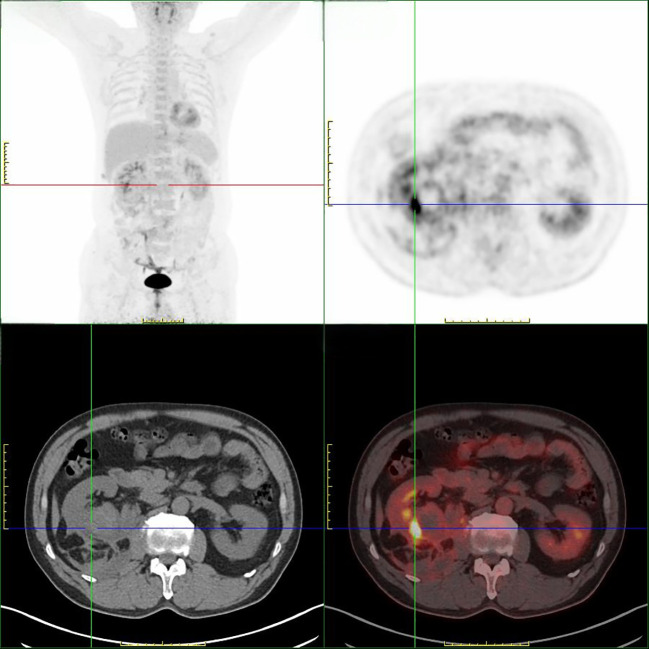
[18F]FDG PET/CT findings. Whole-body maximum-intensity-projection (MIP) image and regional axial CT, PET, and fused PET/CT images of the abdomen. The fused abdominal images show a focal area of increased [18F]FDG uptake posterior to the lower pole of the right kidney with an indistinct interface with adjacent structures, consistent with a neoplastic lesion with imaging features suggestive of rupture.
